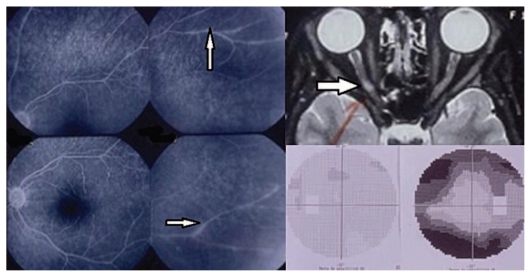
Monosymptomatic optic neuritis (MSON). Top right shows an MRI image of a demyelinating plaque of the right optic nerve with corresponding visual field changes (bottom right). Retinal vasculitis is well evident on fluorescein angiography (left frames, arrows).
